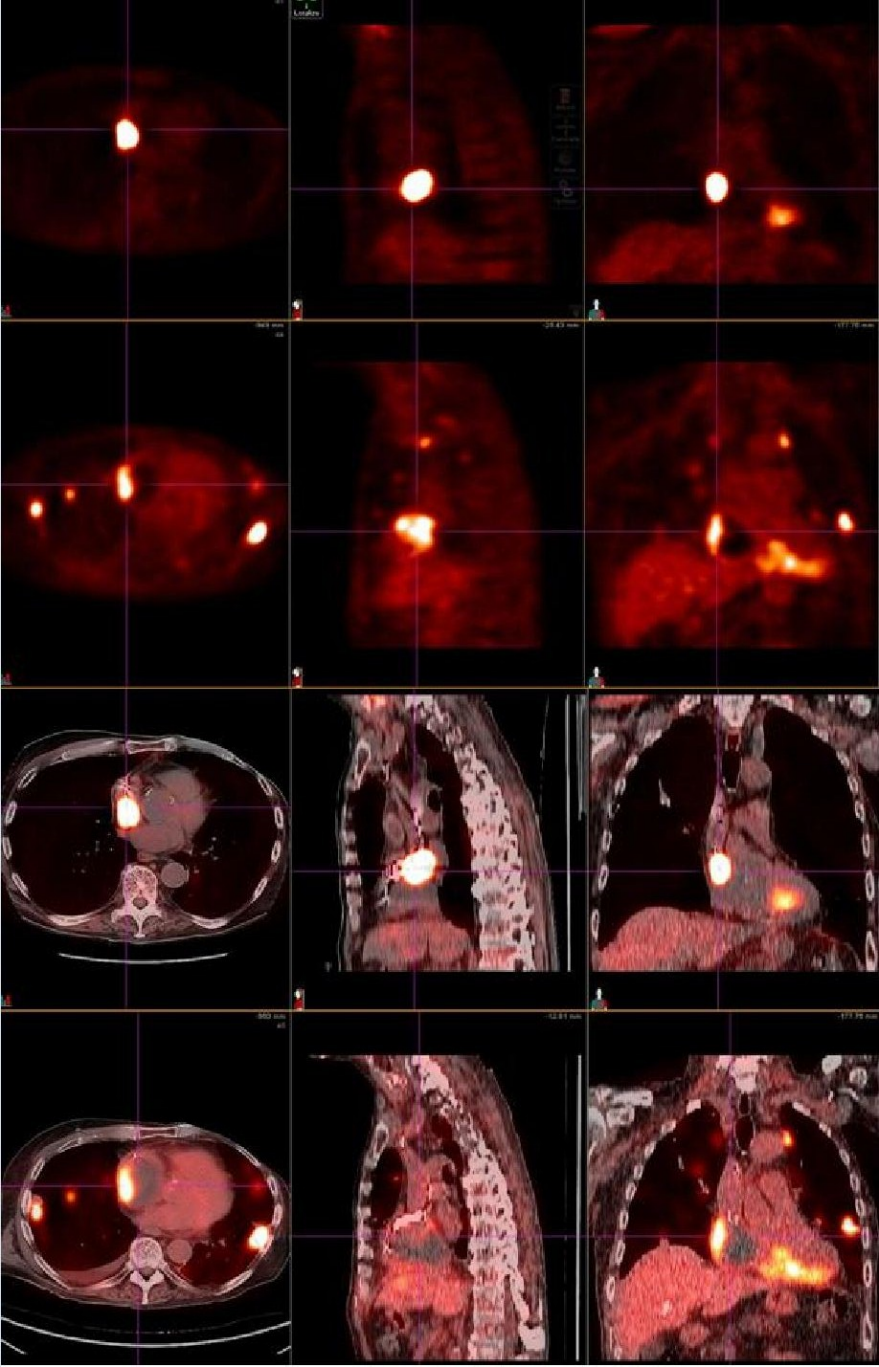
PET Response to treatment of an intracardiac metastases in the R ventricle and atrium. The top panel demonstrates a PET-CT scan of the hypermetabolic tumor mass prior to treatment on June 8 2009; the lower panel shows the tumor mass with notably decreased FDG uptake after treatment on August 30 2009.
